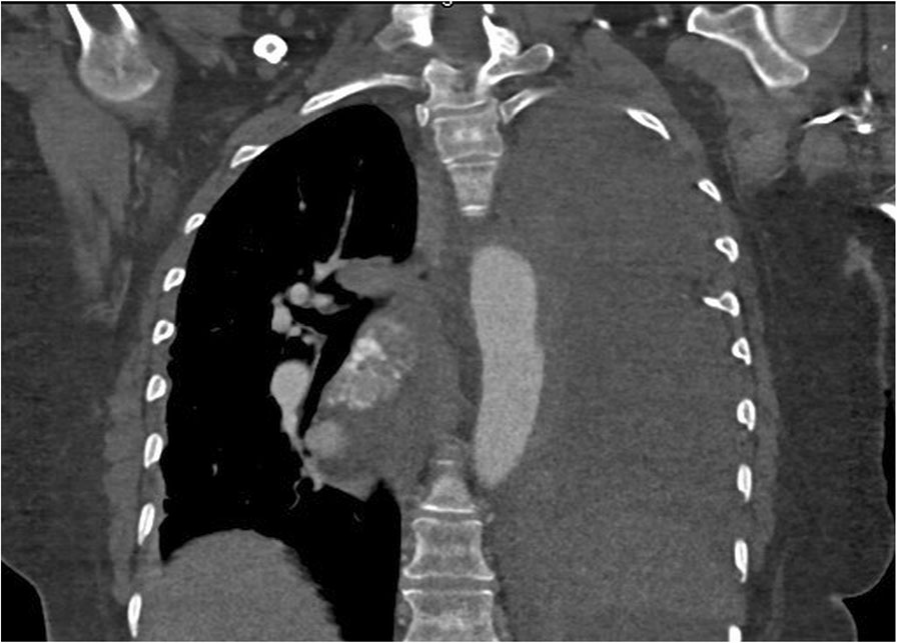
Pre-TEVAR CTA (coronal view) showing focal intimal irregularity in the distal aortic arch. No evidence of active extravasation
